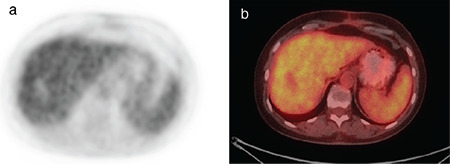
^18^F-FDG-PET/CT of a patient with complete response to the treatment of a liver metastasis of breast cancer 2 months post RFA. The photophenic zone in the 4b liver segment on the metabolic images corresponds with coagulation necrosis. a) PET post-treatment image, axial slice on the level of the 4b segment of the liver, b) fused axial slice on the level of the 4b segment of the liver, post-treatment image ^18^F-FDG: ^18^Fluorine-fluorodeoxyglucose, PET/CT: Positron emission tomography/computed tomography, RFA: Radiofrequency ablation
